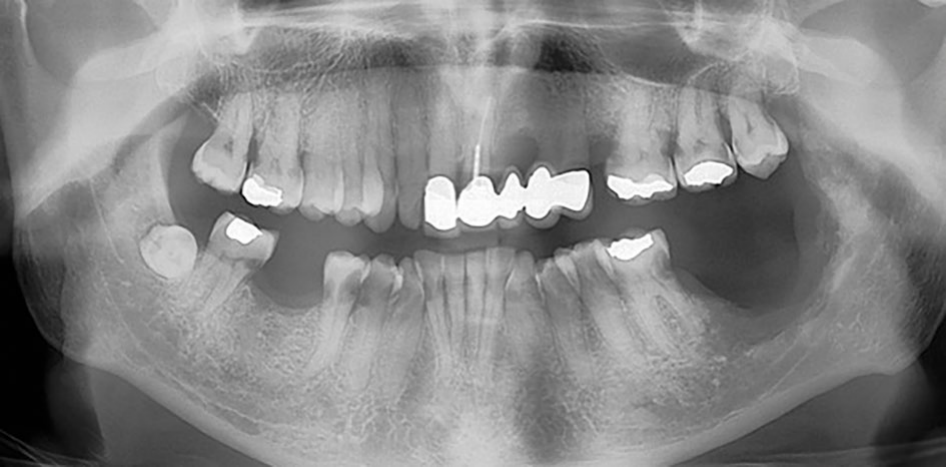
Dental panoramic radiograph for the same patient only 15 months after the completion of radiotherapy showing widespread radiation caries and osteoradionecrosis to the left posterior mandible
